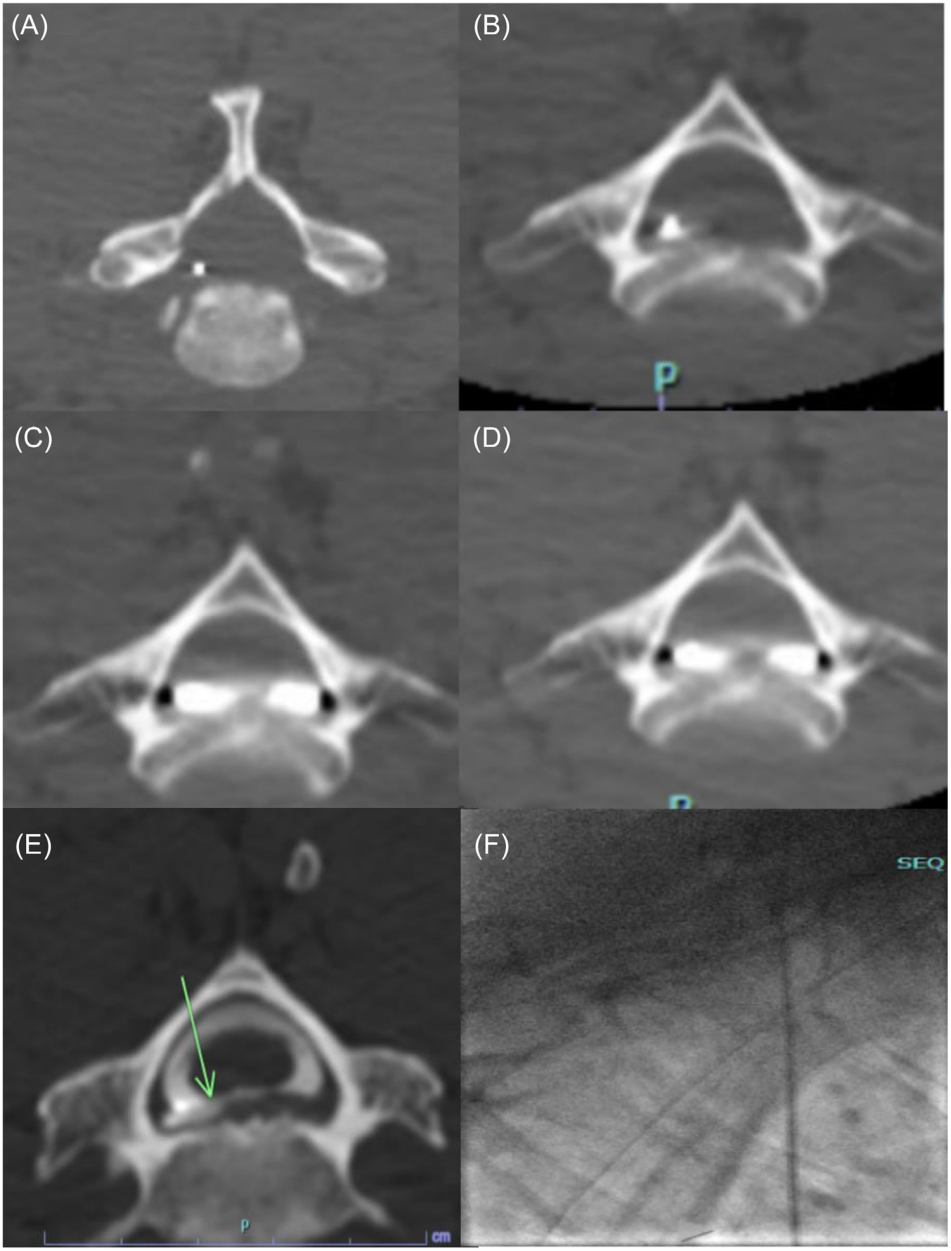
Dynamic CT myelogram demonstrating the opacification of the thecal sac at the distal tip of the microcatheter representing how quickly opacification evolves over seconds potentially hindering the ability to detect site of CSF leak. A, displays the site before injection; B, C, and D were captured at 30 second intervals after contrast injection. E, shows the focal CSF leak origin compared with (F), which shows best cervical visualization during DSM in prone position. CSF indicates cerebral spinal fluid; CT, computed tomography; and DSM, digital subtraction myelography.
